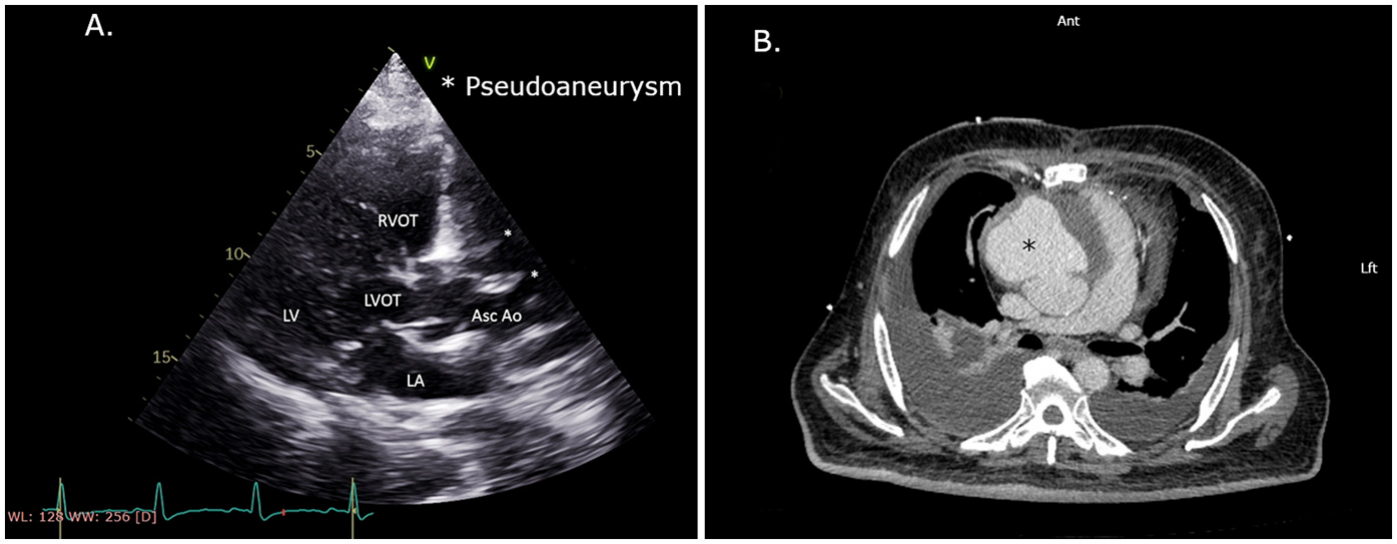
(A) Echocardiography. PLAX view with a decrease in left ventricular function and suspected pseudoaneurysm of the ascending aorta. (B) CTA scan with an anterior ascending aorta pseudoaneurysm showing a mass effect on the heart and pulmonary arteries. CTA, computed tomography angiography; PLAX, parasternal long-axis.
